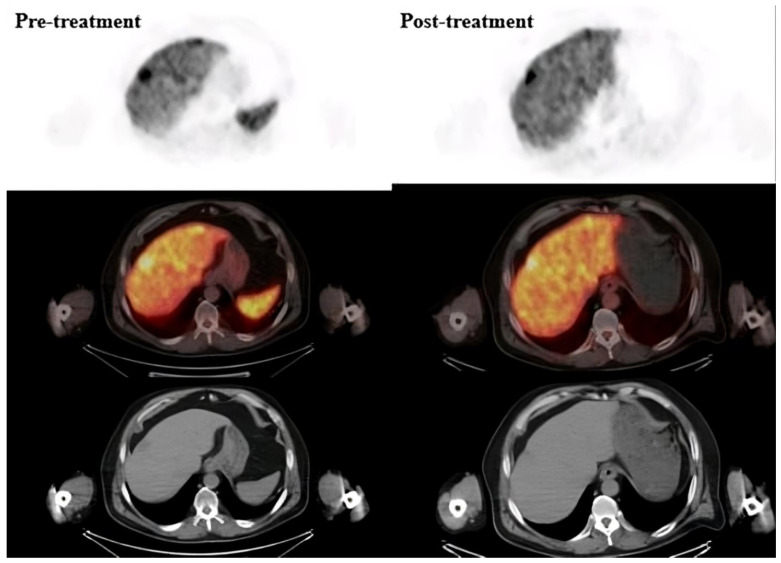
The pre-and post-treatment Ga-68 PSMA PET/CT images of a representative patient. The pre-treatment image shows liver lesions with high PSMA uptake (SUVmax: 60–68), indicating significant metastatic involvement. The post-treatment image, obtained after two cycles of Lu-PSMA-617 therapy, demonstrates a reduction in both lesion size and molecular activity, with a corresponding decrease in SUVmax values (SUVmax: 30–35).
